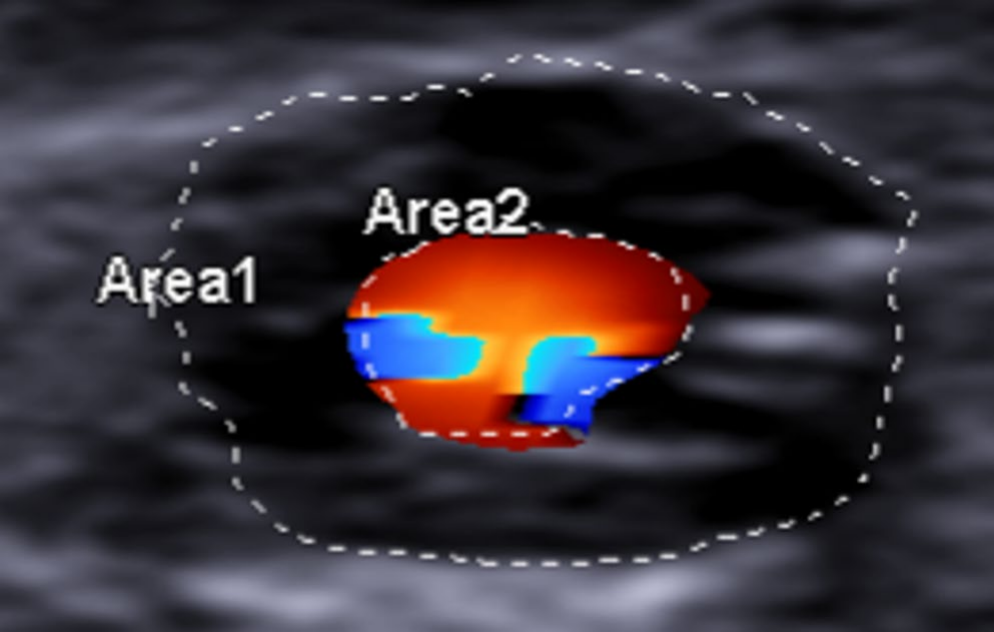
Transverse scan. Color Doppler ultrasound revealed turbulent flow in the vessel segment exhibiting an 83% obstruction due to an atherosclerotic plaque
